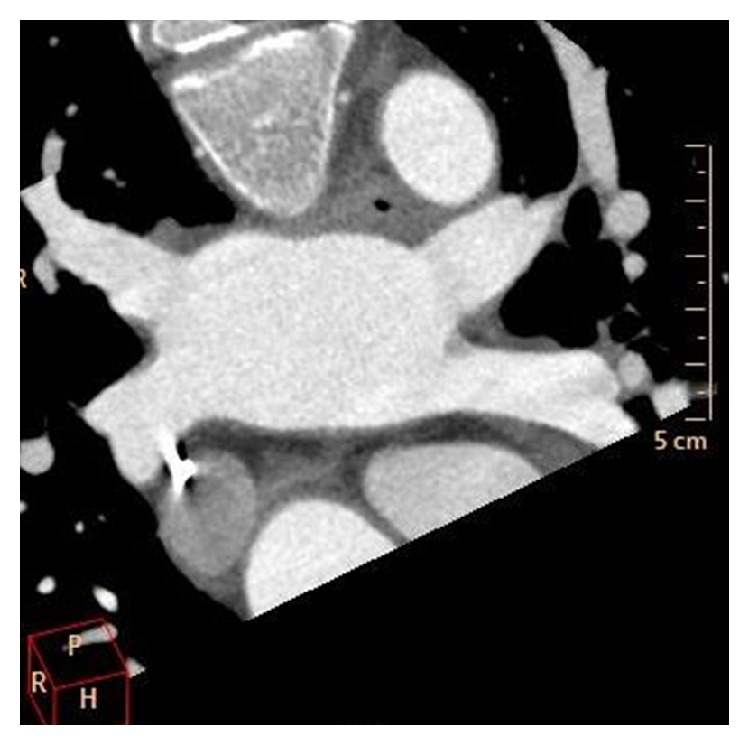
Contrast-enhanced CT scan showing two left-sided and two right-sided pulmonary veins.
